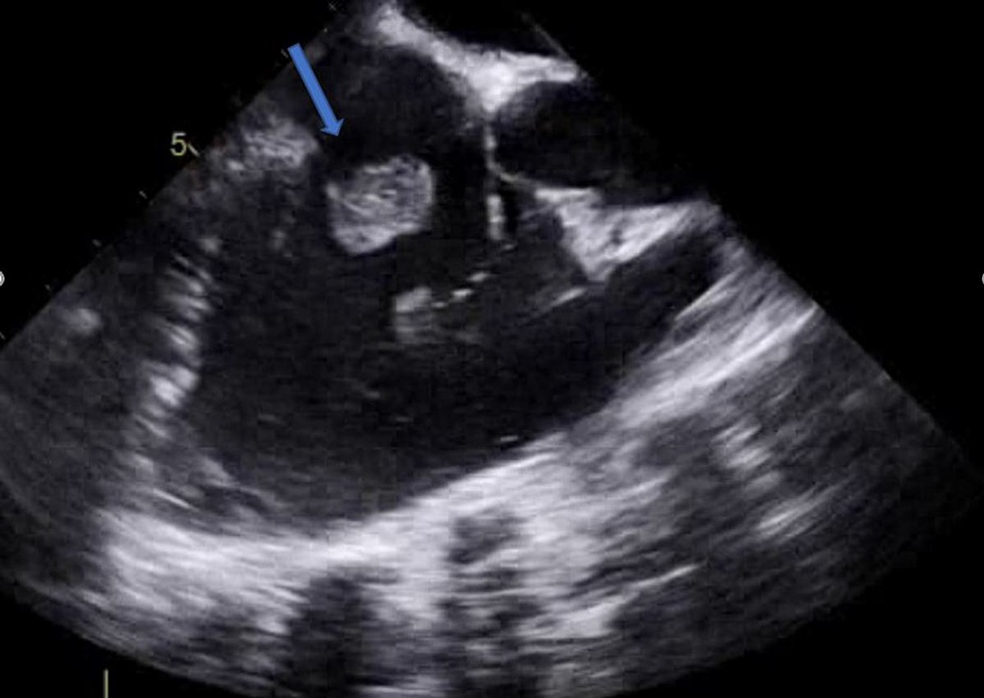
Transesophageal echocardiogram three-chamber mid-esophagus view Blue arrow showing tricuspid valve vegetation
